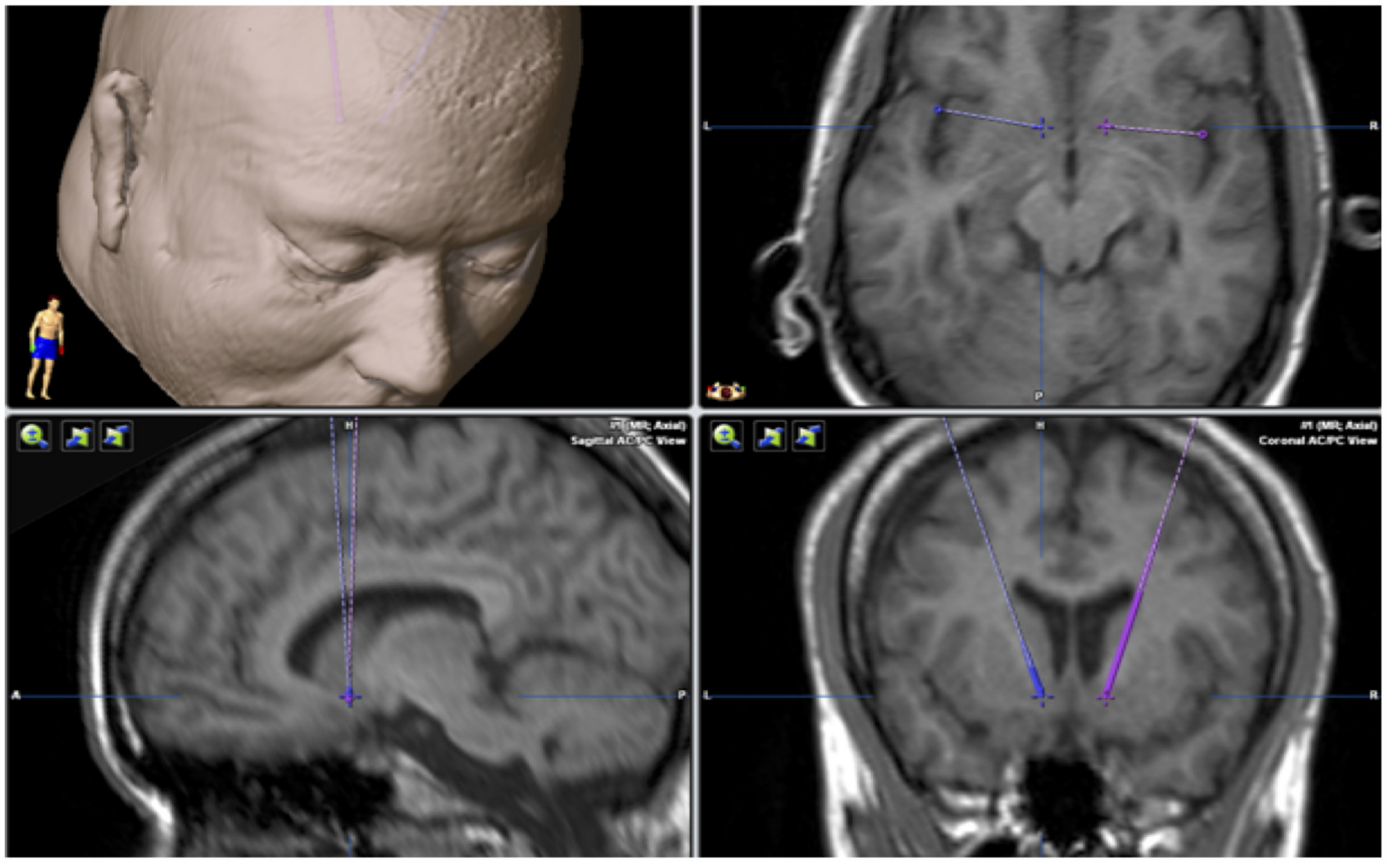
Stereotactic planning. MRI examination with a planned target in the nucleus accumbens in 3 planes. The cross points to the target, and the solid and dashed lines indicate the course of the electrodes in the brain.
